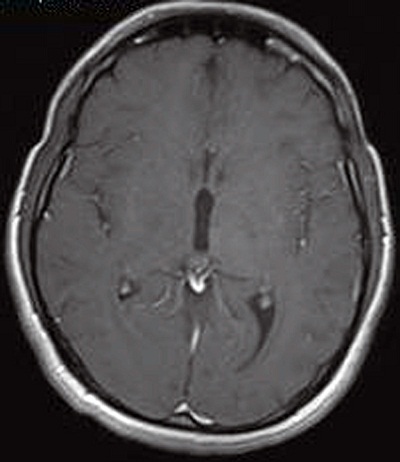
T1-weighted axial MR image with contrast four months after CT-guided stereotactic biopsy and antibiotic therapy, showing a substantially reduced abscess volume and a residual encapsulated area
